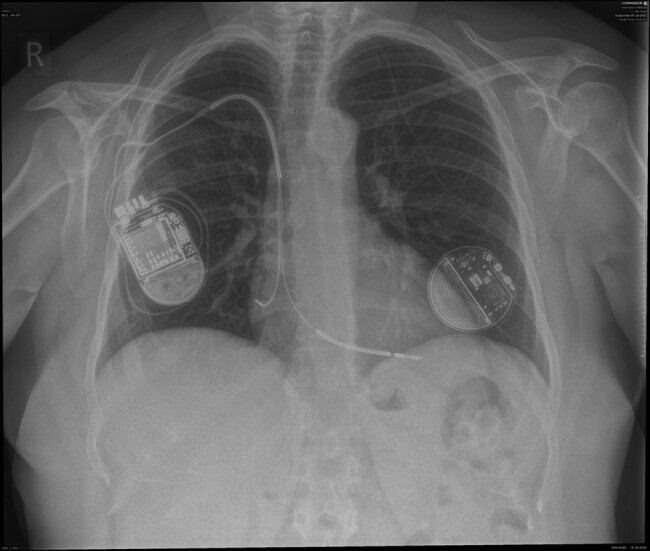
Chest X-ray shows a right-sided transvenous dual-chamber implantable cardioverter defibrillator (ICD) and a left-sided vagus nerve stimulation (VNS) device.
